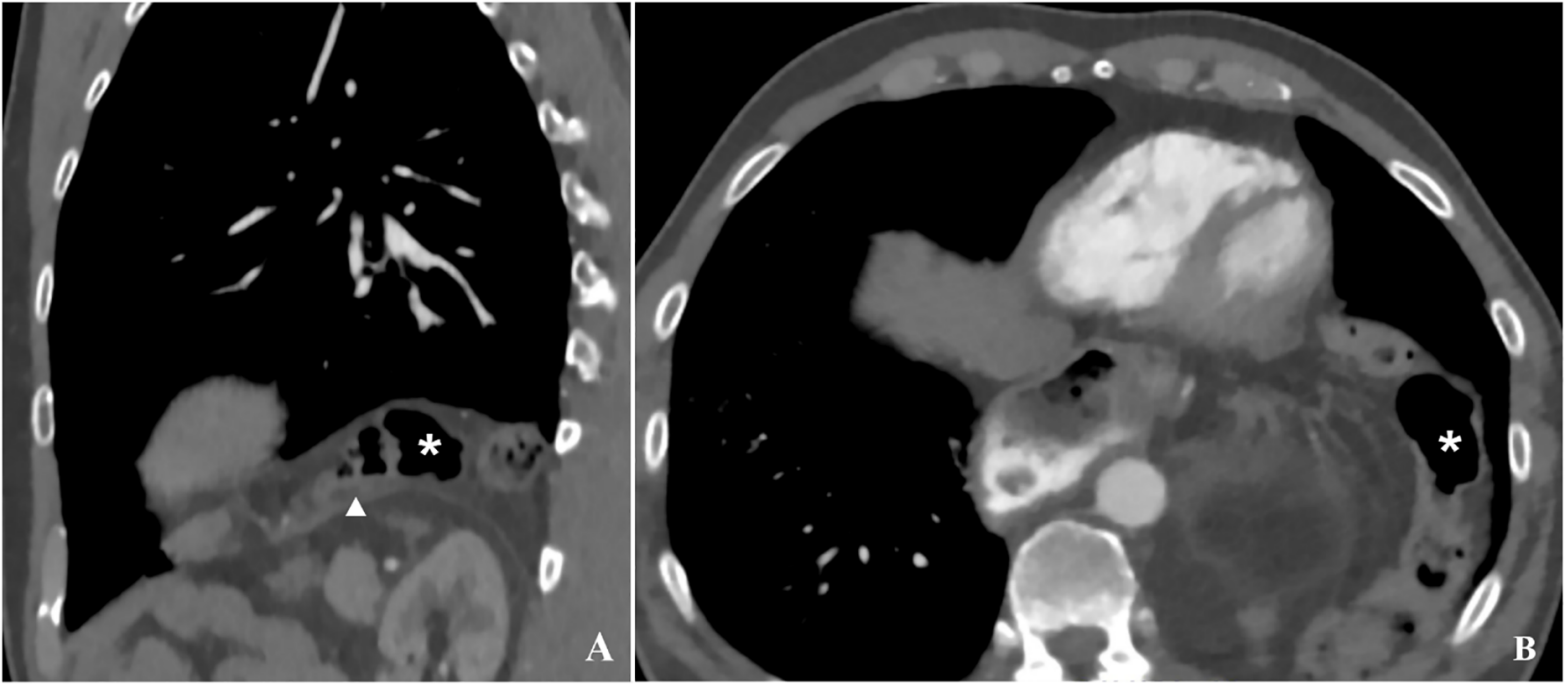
Latest available follow-up CT imaging prior to the patient presenting with acute symptoms in sagittal (A) and transverse (B) reconstruction. At the base of the thorax, the transverse colon (star) can be seen directly above the diaphragm, suggesting a larger scale hernia. This is difficult to see in axial reconstructions (B), however it becomes evident in sagittal reconstructions (A). The triangle on image B marks the left hemidiaphragm.
